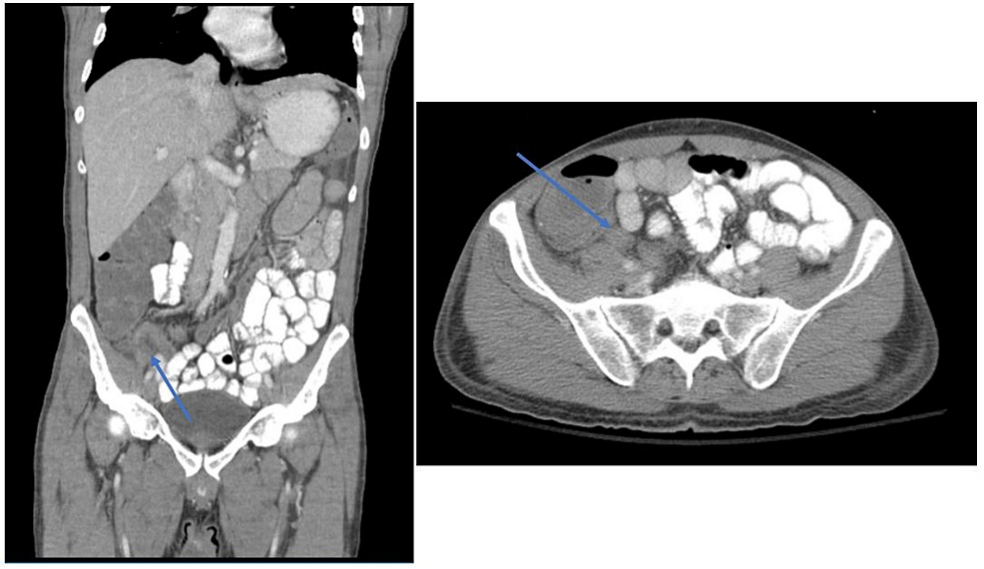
Repeat computed tomography (CT) abdomen and pelvis with contrast Blue arrows represent a mildly thickened appendix with trace inflammatory changes.
